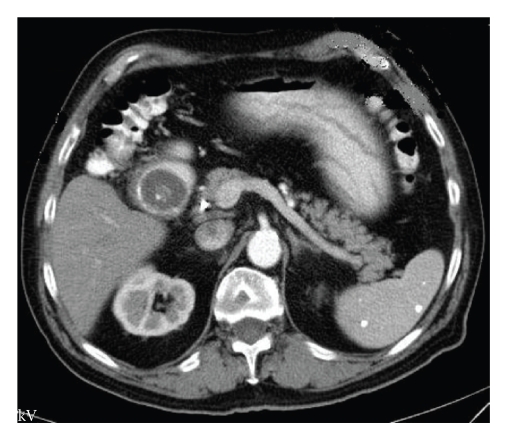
A gallstone in the duodenum and a distended stomach was seen on CT scan.
